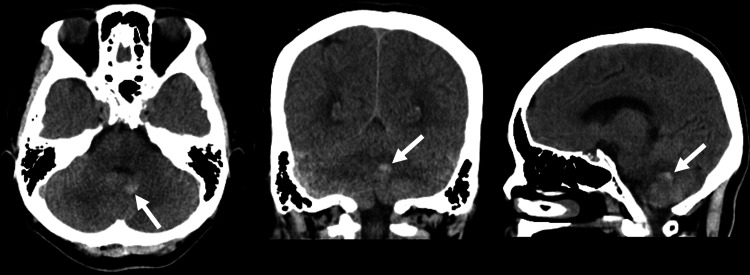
Head computed tomography revealing a slight hemorrhage (white arrow)
